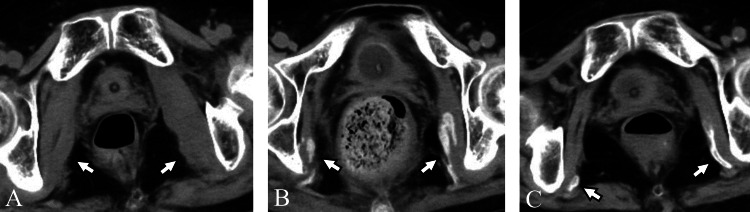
Case one A 72-year-old woman with myositis ossificans in the bilateral short external rotator muscles. (A) On the 13th day of hospitalization, CT revealed swelling in the muscles (arrows). Manual muscle testing score in both lower limbs was 0. (B) On the 27th day of hospitalization, CT performed to evaluate gastrointestinal bleeding showed calcification within the bilateral muscles (arrows), with a decrease in the swelling. The fever had resolved. (C) On the 83rd day of hospitalization, CT for investigation of the fever source revealed ossification within the bilateral muscles (arrows).
